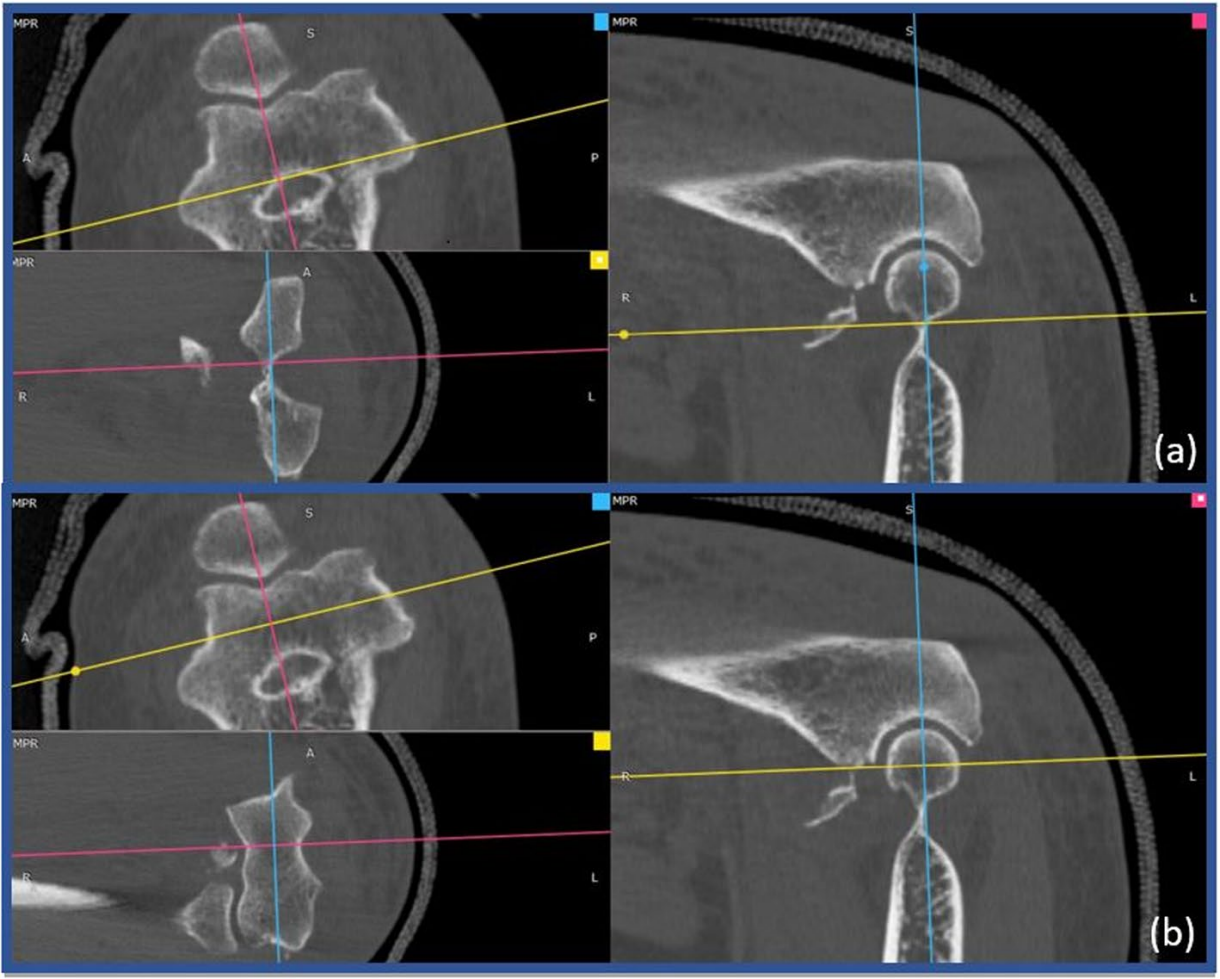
CT Standardization - 3D MPR. (a) Position the blue line parallel to humeral diaphysis in sagittal view and parallel to the transepicondylar axis in axial view. Locate the yellow line parallel to the trochlear axis in coronal view. Place the pink line passing through the deepest point of the trochlea in axial and coronal view. (b) Position the intersection between the blue line and the yellow one in the center of the humeral trochlea in sagittal view
